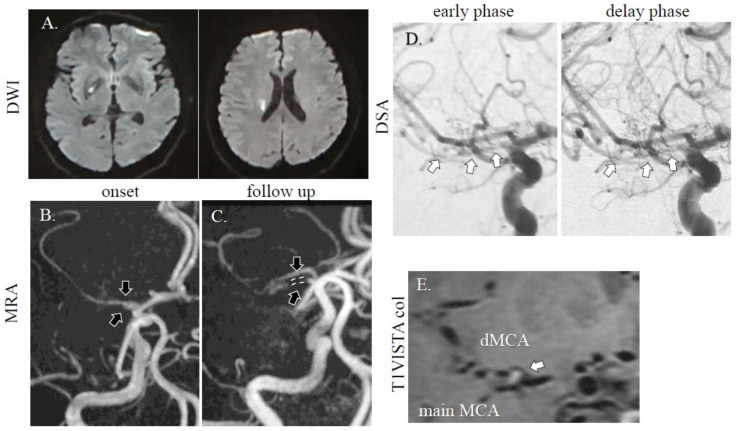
MRI/A and DSA images of case 2. A dotted high-intensity area was observed in the right basal ganglia area (A). The right MCA was faint (B; downwards arrow: intact MCA, upwards arrow: stenotic MCA), and there was one intact MCA and one stenotic MCA (C; downwards arrow: intact MCA, upwards arrow and dotted line: stenotic MCA). On DSA, a duplicated MCA was observed (D; arrows: stenotic MCA). On T1-VISTA colonal (col) image, intramural hematoma was found in the origin of duplicated MCA (E; arrow). DWI; TR, 7000 ms; TE, 120 ms. MRA (without using contrast agent); TR, 30; TE, 6.8 ms. T1-VISTA; field-of-view (FOV): 120 mm, TR: 400 ms, TE: 10ms, volume-of-interest (VOI) size: 1 × 1 × 1 mm, 100 slices.
